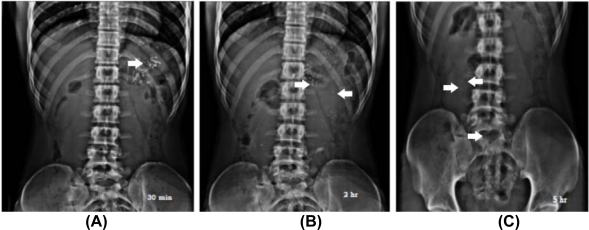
X-ray photographs recorded at (A) 0.5 h, (B) 2 h and (C) 5 h after oral administration of blank formulation of ORZ-6 in human volunteers.
